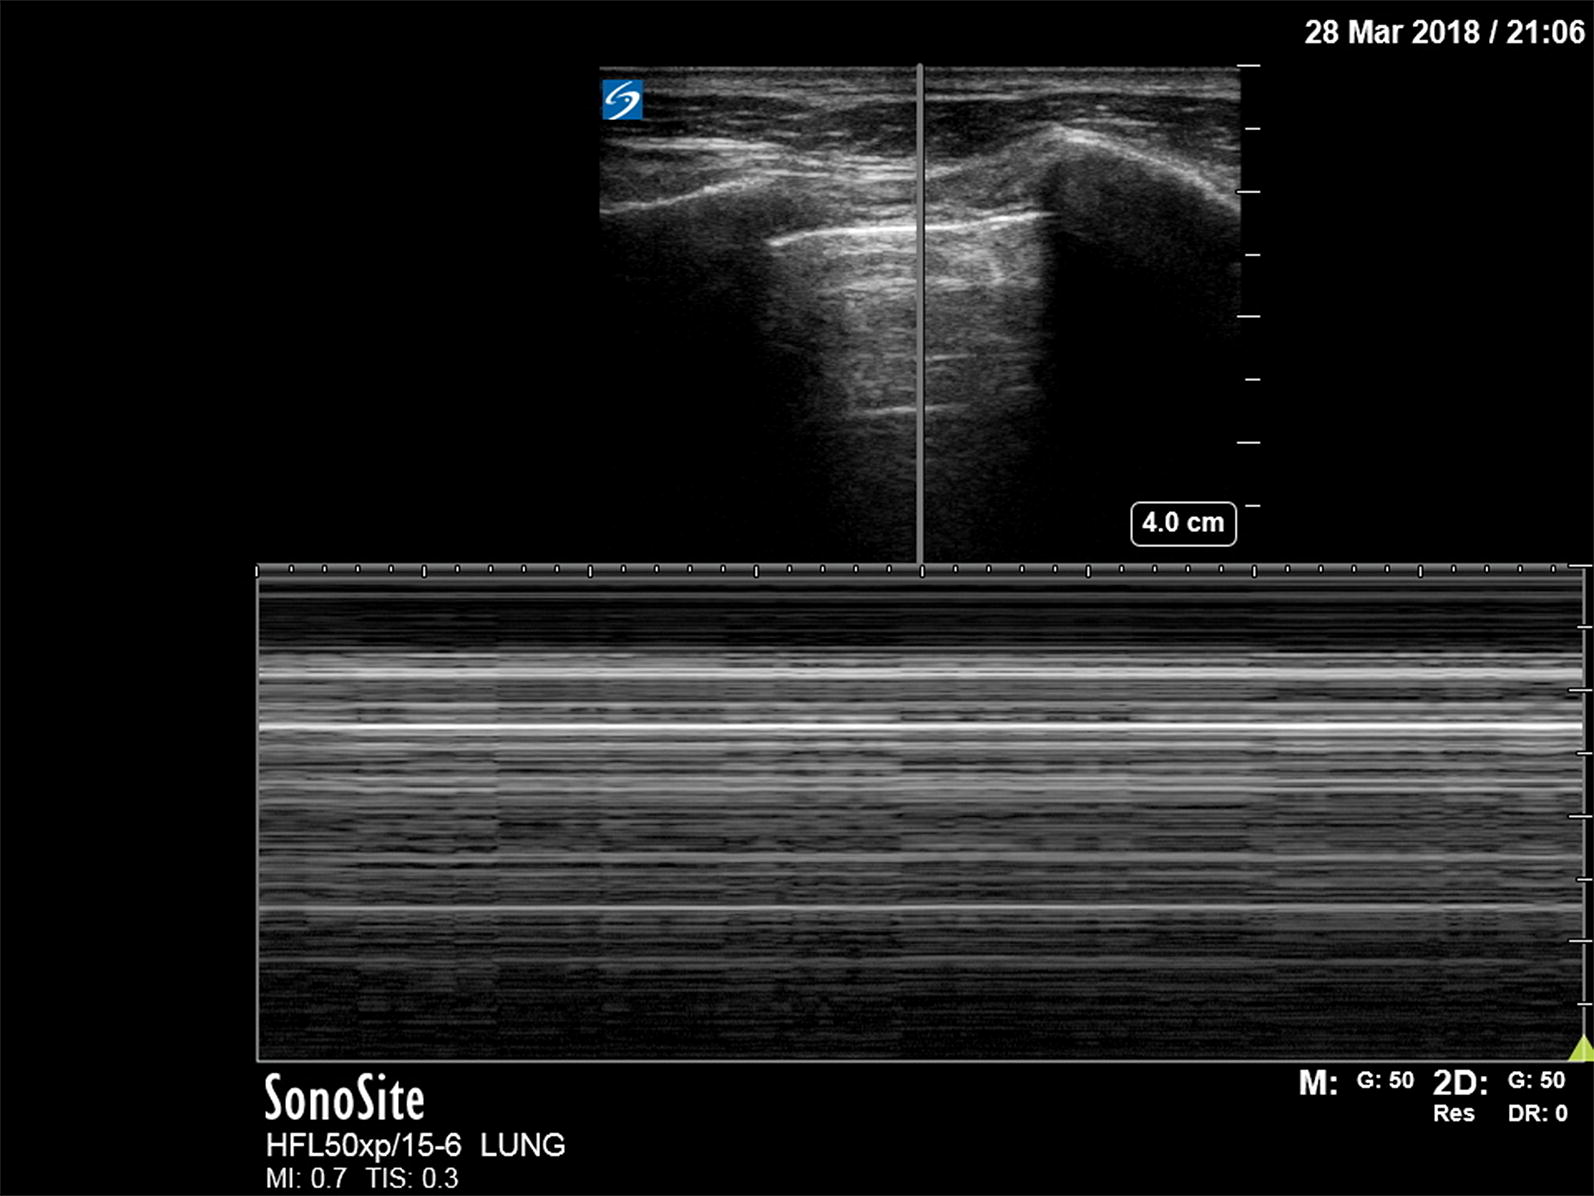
Pneumothorax—M-mode ultrasound image. There is no visible movement above or below the pleural line. Because all tissue and artifacts are stationary, the M-mode image appears as horizontal straight lines throughout the image. This is called a barcode sign or stratosphere sign
